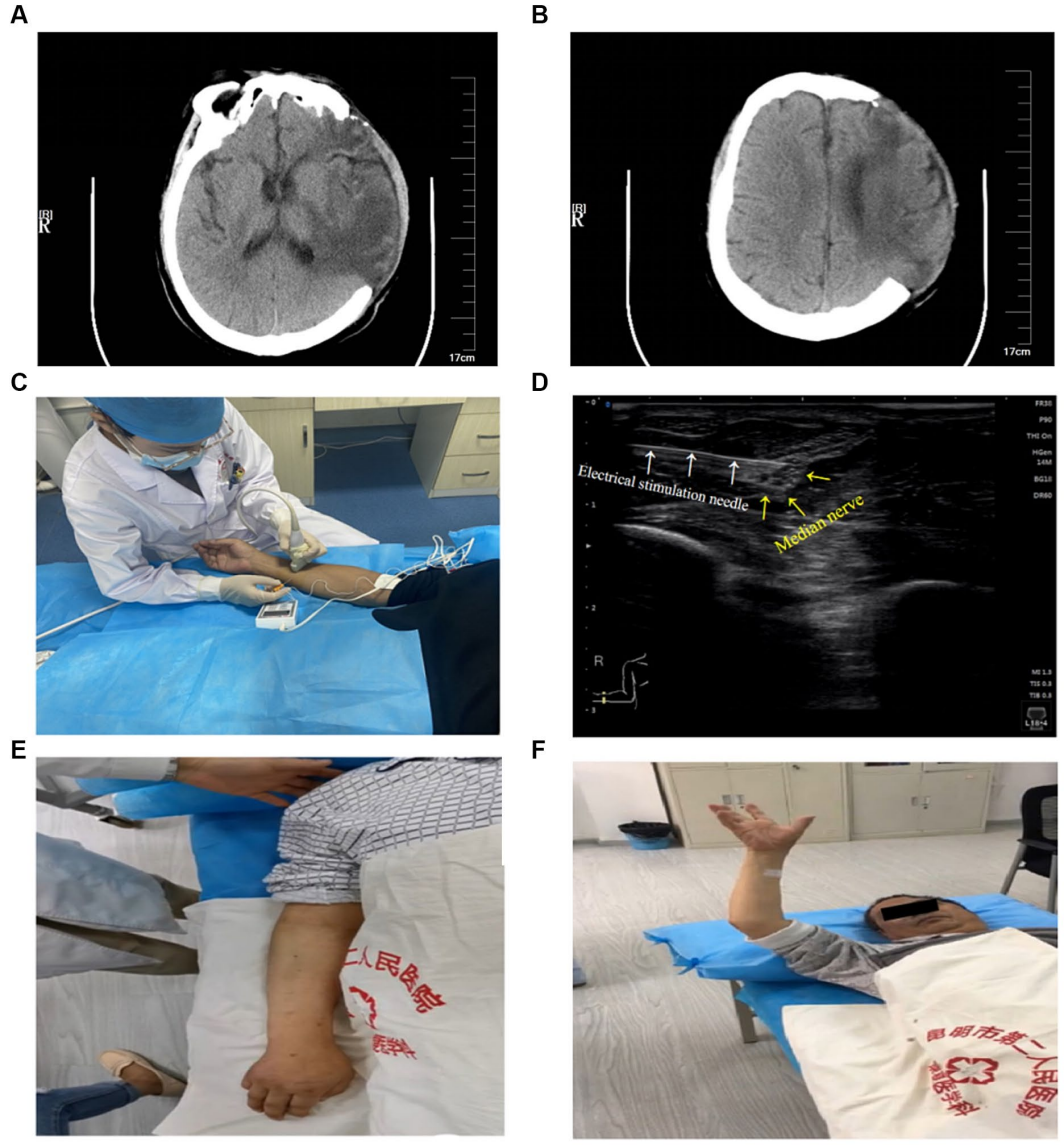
Head CT scan 2 weeks after surgery. (A) Dorsal thalamic level: irregular cerebral edema in the left frontal lobe, temporal lobe, and posterior limb of the internal capsule, and meningoencephalocele after the left frontotemporal parietal bone flap removal. (B) Lateral ventricle body level: irregular cerebral edema in left frontal and parietal lobes, and meningoencephalocele after left frontotemporal parietal bone flap removal. UG-MNES treatment and upper limb function. (C) Application of right median nerve electrical stimulation under ultrasound guidance. (D) Ultrasound image of electrical stimulation needle (white arrows) and median nerve (yellow arrows). (E) No casual movement of the right upper limb before the treatment. (F) Immediate flexion and extension of the hemiplegic upper limb and fingers after the first treatment.
